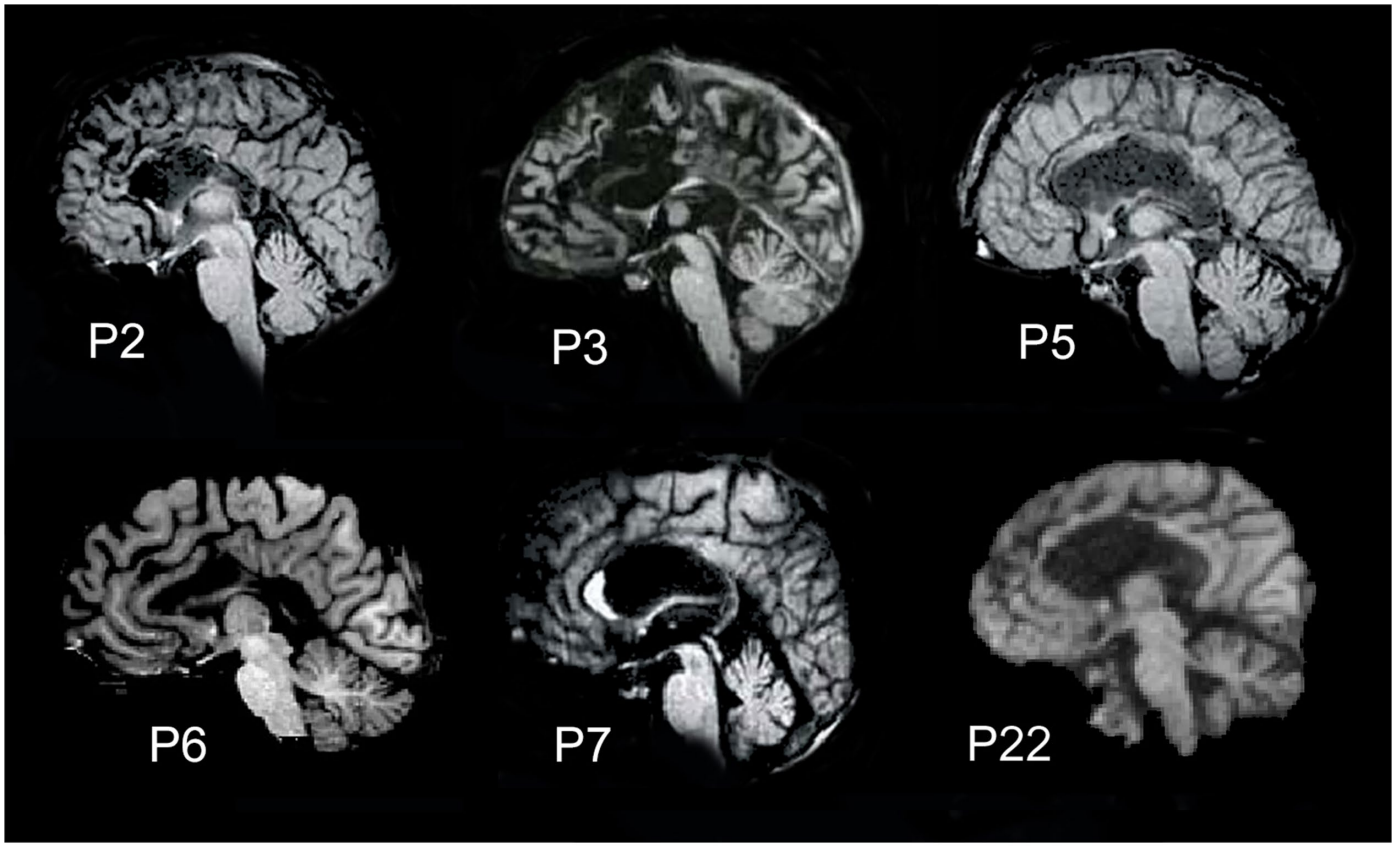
Midsagittal magnetic resonance images showing the extent of callosal resection. Five patients (P2, P3, P5, P6, and P22) carried total callosal resection; patient P7 had a partial posterior resection.
